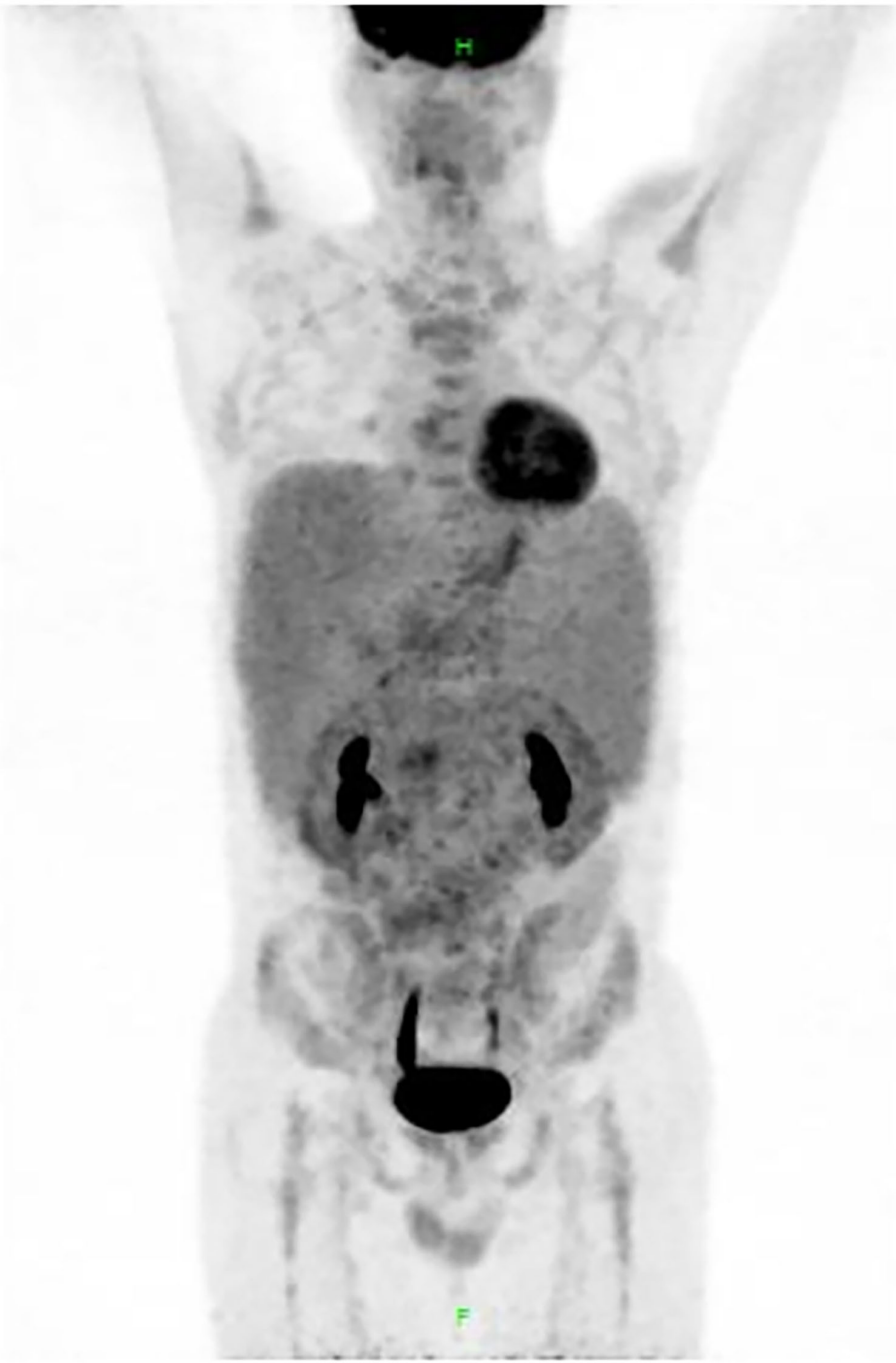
Results of positron emission tomography (PET)-CT. PET-CT scan revealed multiple lymphadenopathy (SUVmax 5.0) in the bilateral cervical, bilateral axillary, mediastinal, retroperitoneal, supra-mesenteric, iliac vessel, and bilateral inguinal region, and the maximum cross section of the lymph node mass was 10.9 cm × 10.2 cm. Liver (SUVmax 3.2) and spleen (SUVmax 3.0) were significantly enlarged. Metabolism of central and peripheral bone marrow was diffusely increased, with SUVmax of 3.6.
